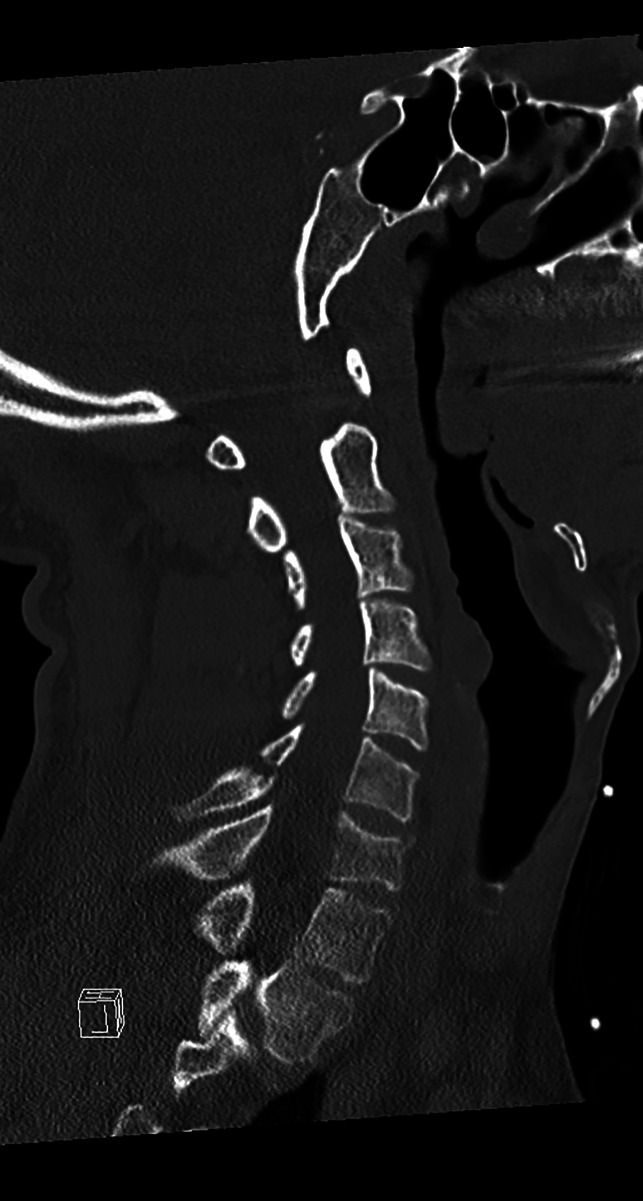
CT scan showing C6 spinous process fracture seen on CT.
